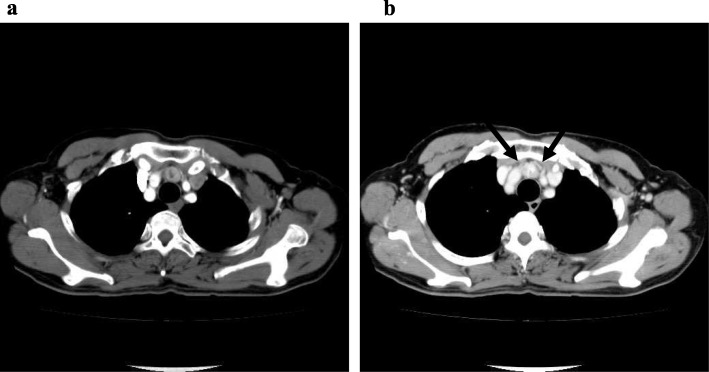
Contrast-enhanced CT images of ectopic mediastinal thyroid tissue. a Poor enhancement with a low density of the central region in the early phase. b Strong enhancement, especially of the central region, in the delayed phase. Arrows show tumor-feeding arteries arising from the vessels in the thorax
